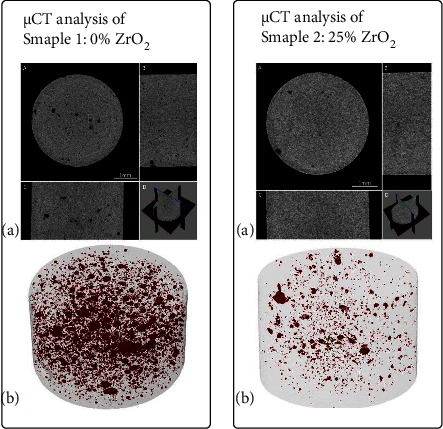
Analysis of the *µ*CT of the set cement: (a) Three perpendicular sections: traverse A longitudinal B and sagittal C along with their spatial visualisation D (b) Visualisation of sample pores in a two-dimensional image.
